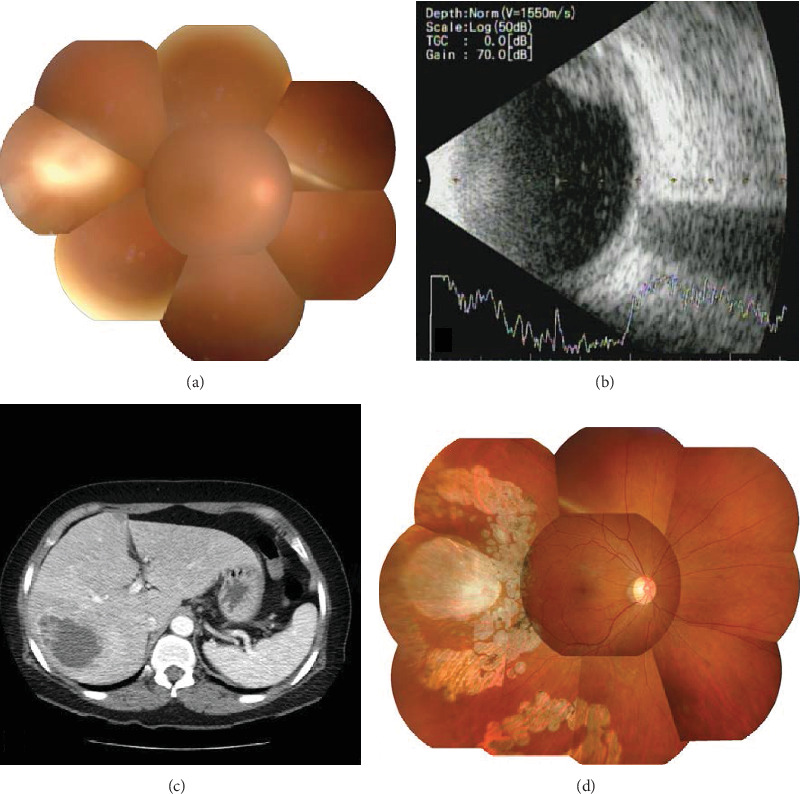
(a) Funduscopy shows a large necrotic lesion in the superotemporal area with vitreous opacity of the right eye. (b) Ultrasound shows abscess formation with a height of 5 mm at the temporal macula (c) Abdominal computed tomography shows a pyogenic liver abscess of the right lobe. (d) Fundus, 18 months after admission showing a resolved abscess and atrophic retina at the original abscess site.
